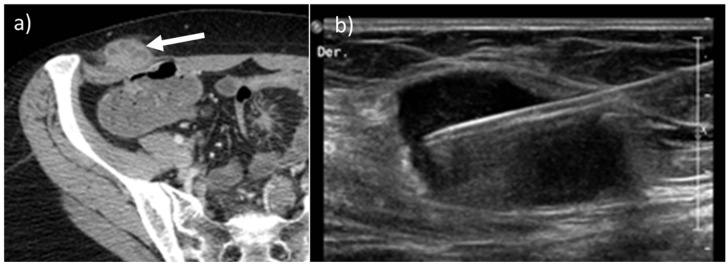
Axial, contrast-enhanced CT (a, arrow) on portal venous phase of a 45-year-old female with history of ovarian cancer treated with laparoscopic surgery demonstrates the presence of a solitary metastatic mass along the right abdominal subcutaneous fat. After laparoscopic surgery, implant tumors can be found along the course of the trocars. Nonetheless, a biopsy was made, as shown on US B-mode picture (b),that confirmed the presence of disease.
